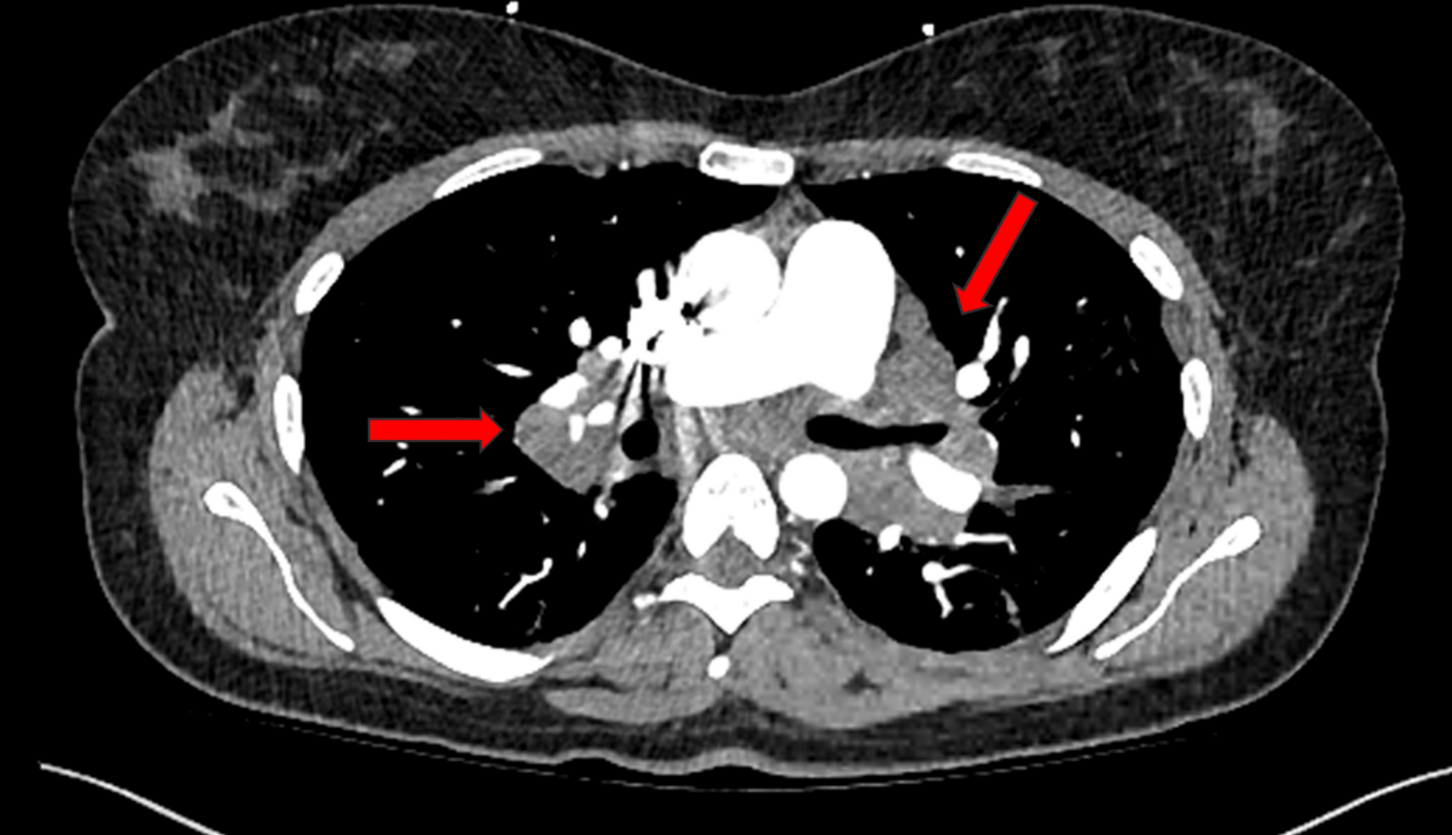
CT of the chest showing enlarged mediastinal and hilar lymph nodes CT: computed tomography
